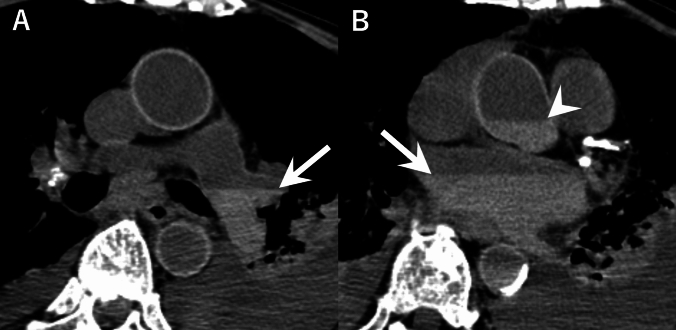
Example of gravitational sedimentation. An 81-year-old woman with acute myeloid leukemia. A Axial postmortem CT showing gravitational sedimentation (arrow) in the pulmonary artery. B Axial postmortem CT showing gravitational sedimentation in the left atrium (arrow) and ascending aorta (arrowhead). CT was performed 155 min after death
